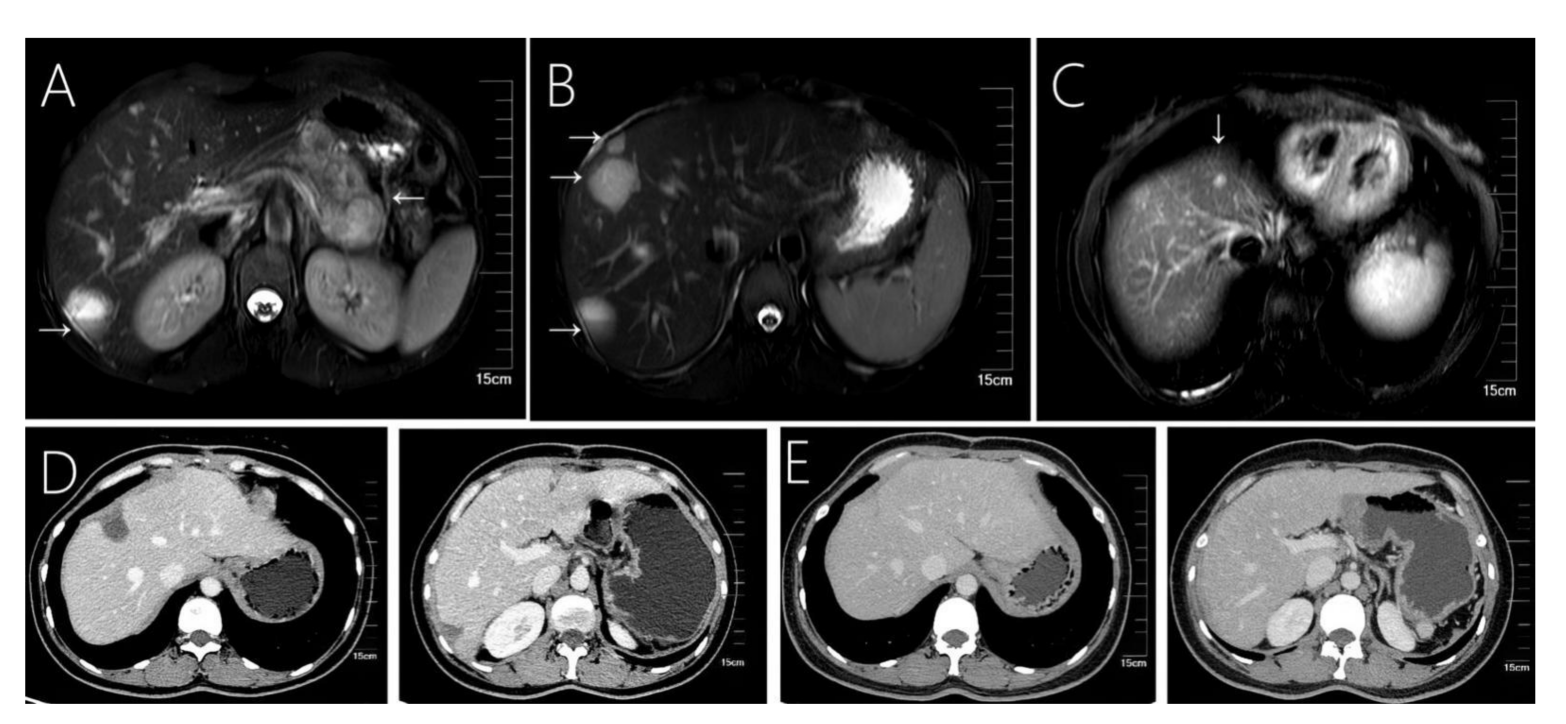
Case 1 shows that 31-year-old female with solid pseudopapillary neoplasm of the pancreas with hepatic metastases. (A) Fast spin-echo T2-weighted image shows a mixed cystic-solid structure within the mass. (B, C) A total of 4 cystic-solid mixed metastases are visible in the liver. (D) A small amount of effusion in the liver operated area was found at the 6th month of follow-up after surgery. (E) Images from follow-up at 36-months, showing a regular liver morphology and no metastatic lesions.
